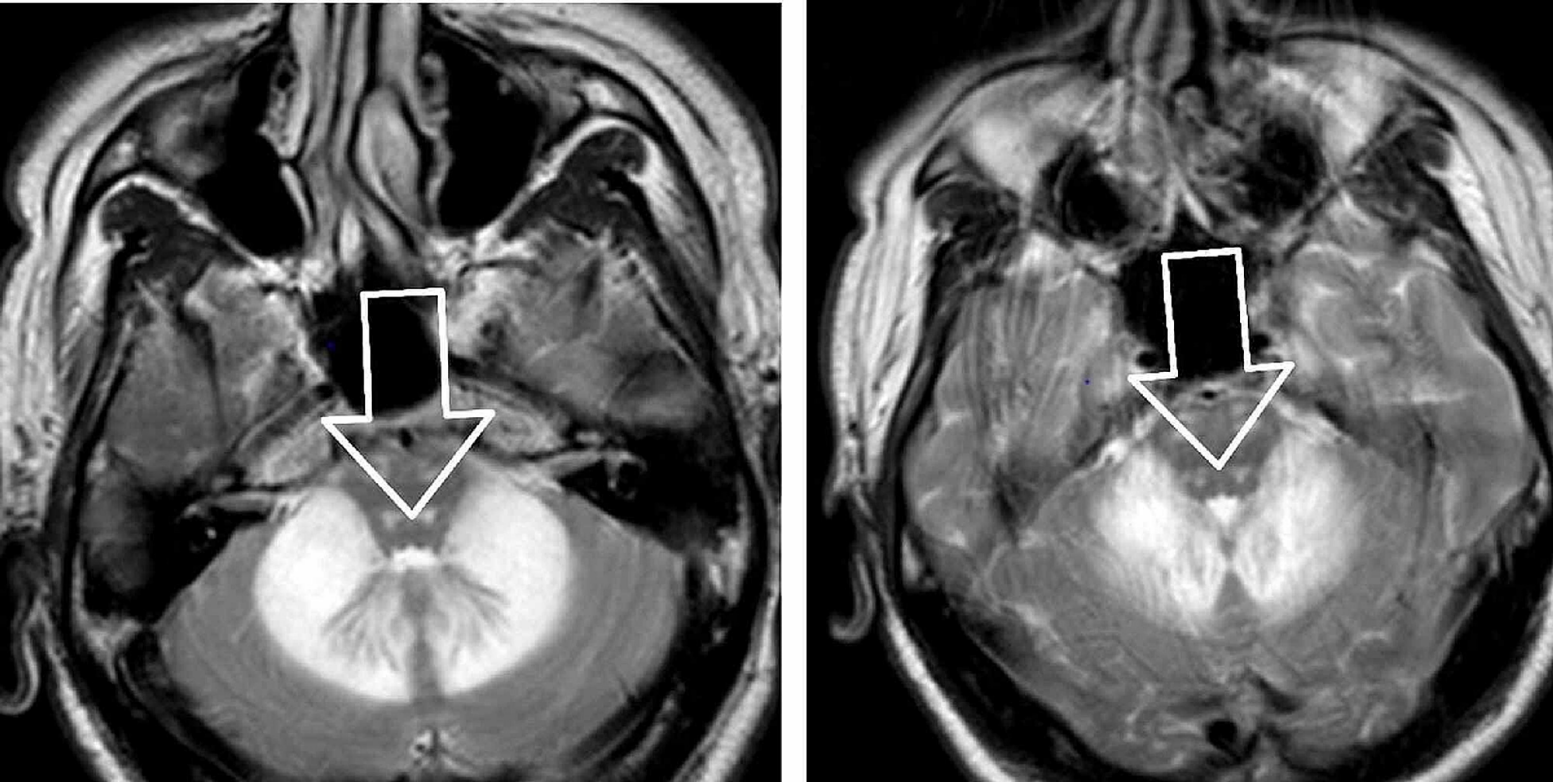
MRI brain without contrast
